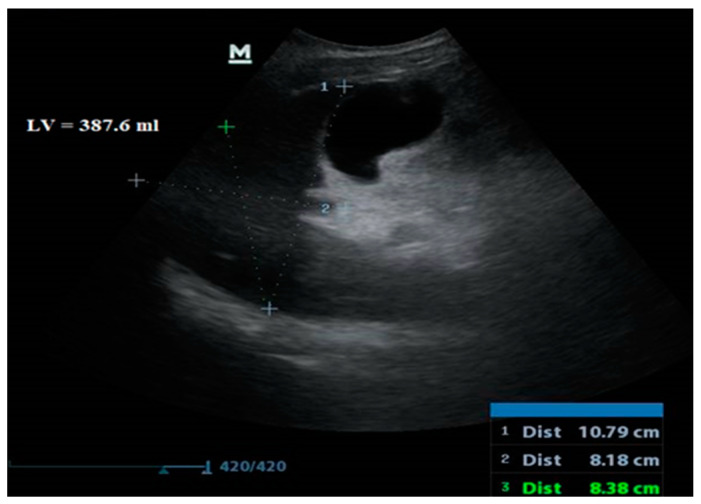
Liver volume measured via abdominal ultrasound.
